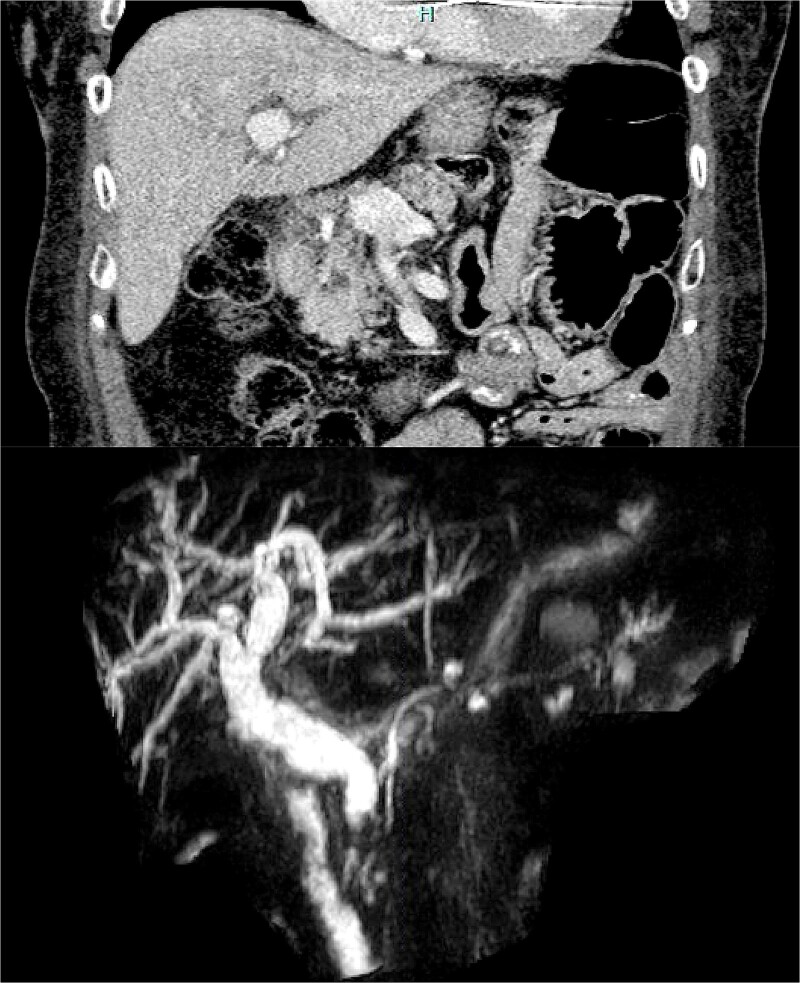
(a) CT scan image of the cystic lesion within the head of the pancreas. (b) MRCP image of intrahepatic and extrahepatic bile duct dilation with abrupt change in caliber at the distal CBD.
